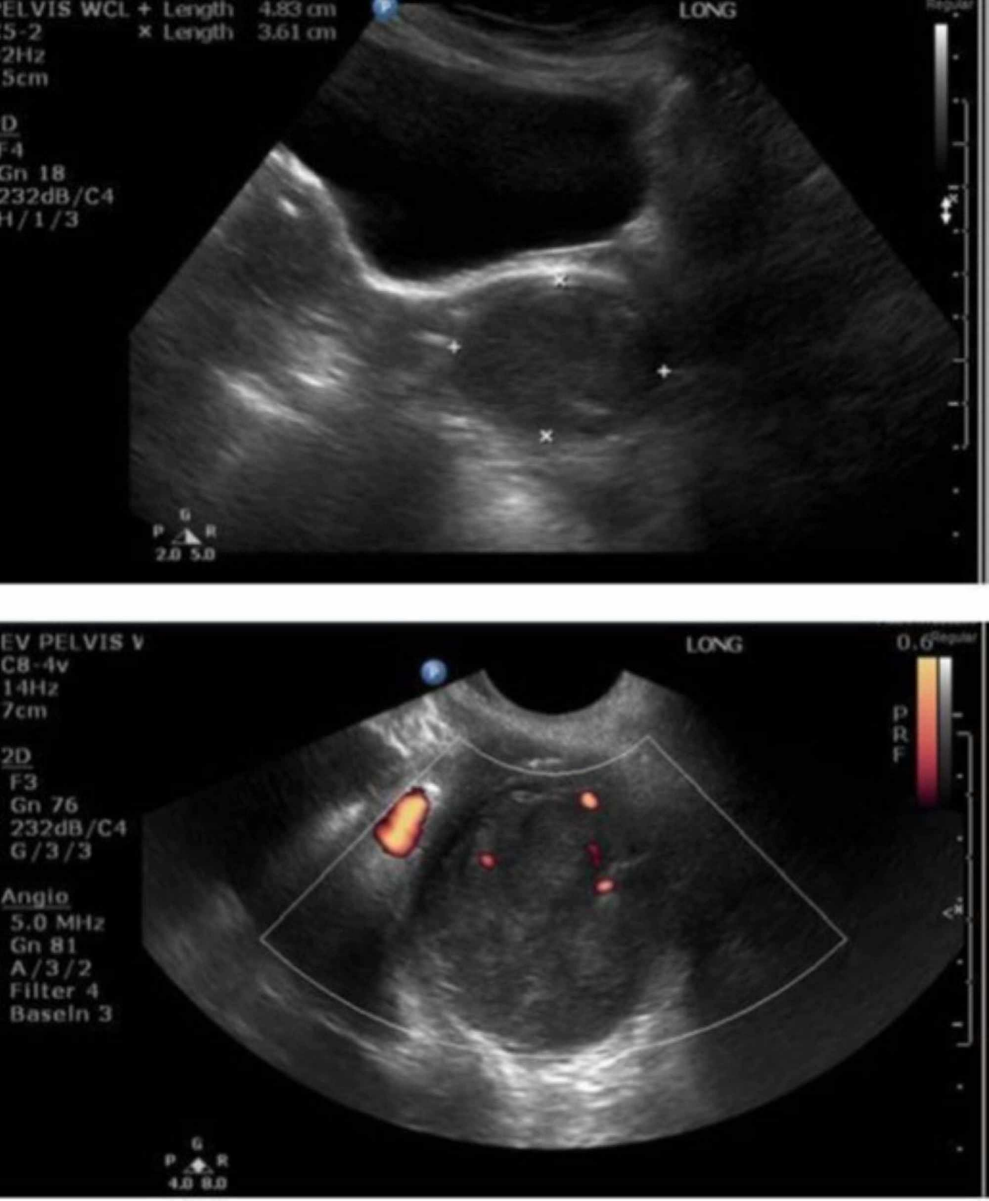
Transvaginal ultrasound with Doppler, showing a 4.8 x 4.4 x 3.6-cm solid mass lesion with internal blood flow, located posterior and inferior to the cervix
